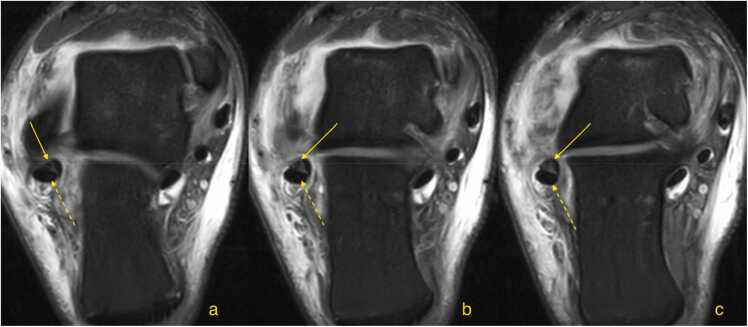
A patient with a recent ankle injury. Magnetic resonance imaging shows an acute peroneus brevis split tear (straight arrow) (a, b, c – oblique cross sections, proton density with fat suppression). The peroneus longus is normal (dashed arrow).
